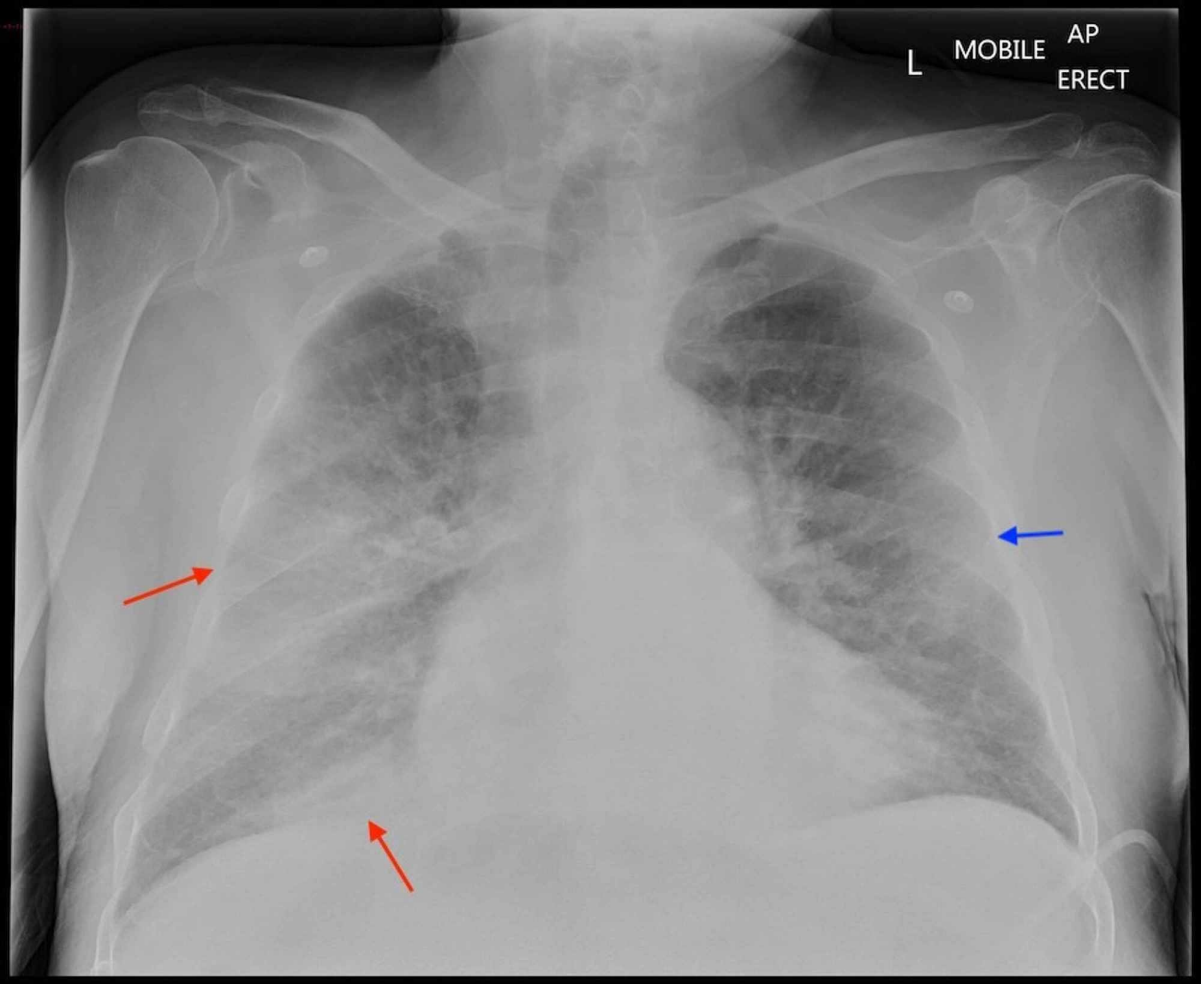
CXR - AP view Ground glass opacification and patchy airspace shadows are seen peripherally in the right middle and lower lung zones (red arrows) and increased attenuation seen peripherally in the left middle lung zone (blue arrow). These features were suspicious of COVID-19 pneumonia. CXR: Chest X-ray; AP: Anteroposterior
